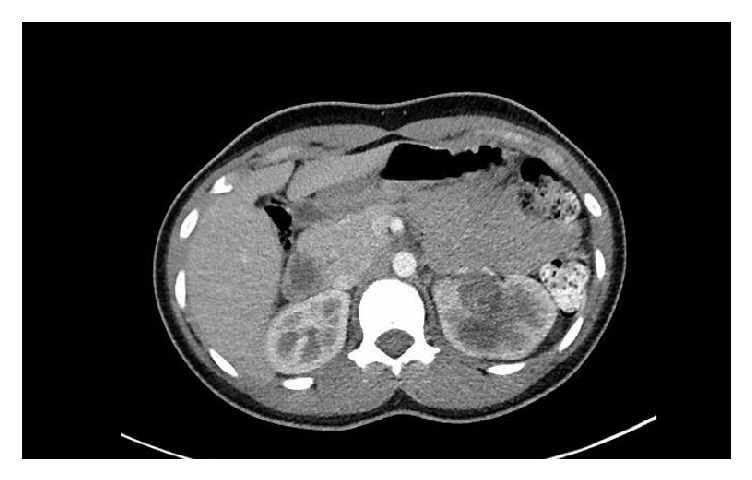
Abdominal computed tomography scan reveals a mass in the left pelvicalyceal system with associated moderate to severe hydronephrosis.
